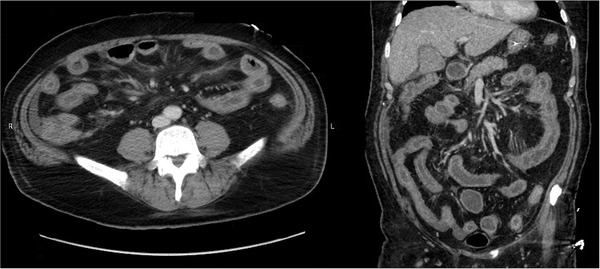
Contrast‐enhanced CT showed mucosal contrast effect and submucosal edema in the entire small intestine and the colon. And there was no decreased contrast effect of the gut suspect of ischemic change or thrombi in the abdominal vessels
